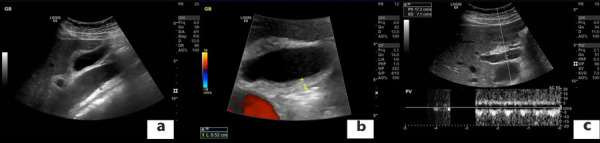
Abdominal ultrasound (US) findings. a, b: Gallbladder edema without gallstones, extra- nor intra-hepatic dilations nor pericholecystic fluid and a dilated inferior vena cava; c: Color doppler sonography demonstrated also reduced blood flow to the inferior vena cava.
